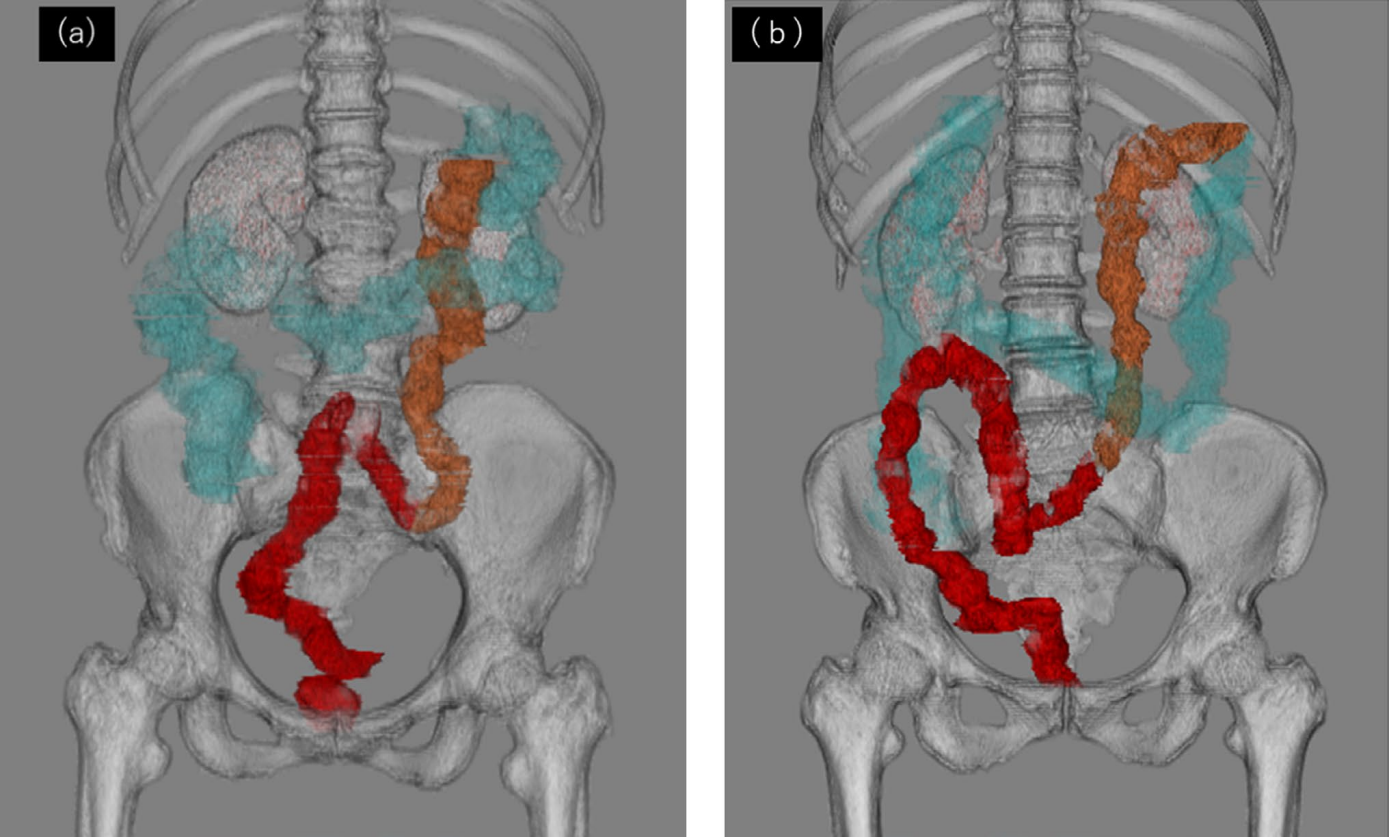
Three‐dimensional computed tomography (3D‐CT) volume rendering image of the colon. (a) Case 1, (b) Case 2: The descending and sigmoid colon are displaced rightward due to persistent descending mesocolon (PDM), making right‐sided exposure of the sacral promontory difficult. Blue: ascending and transverse colon; Yellow: descending colon; Red: sigmoid colon and rectum.
